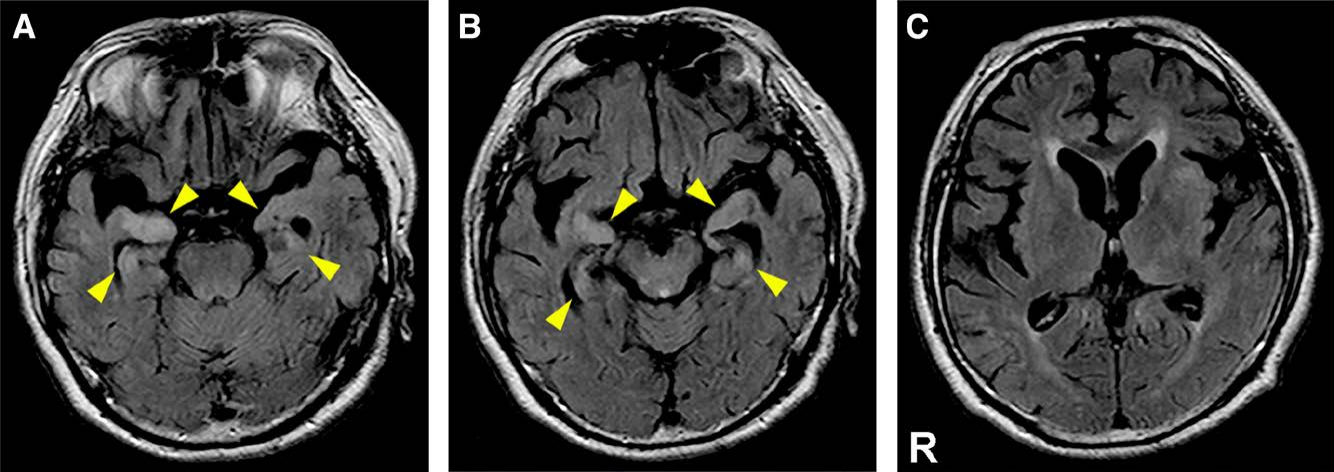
Axial section of T2-FLAIR brain MRI upon admission. (A, B) T2-FLAIR brain MRI upon admission revealed localized high-intensity lesions in the bilateral (highlighted in the right side) medial temporal lobes (indicated by yellow arrows heads). (C) Abnormal signals were not observed in other limbic area such as the insula. MRI = magnetic resonance imaging, T2-FLAIR = T2-weighted fluid-attenuated inversion recovery.
